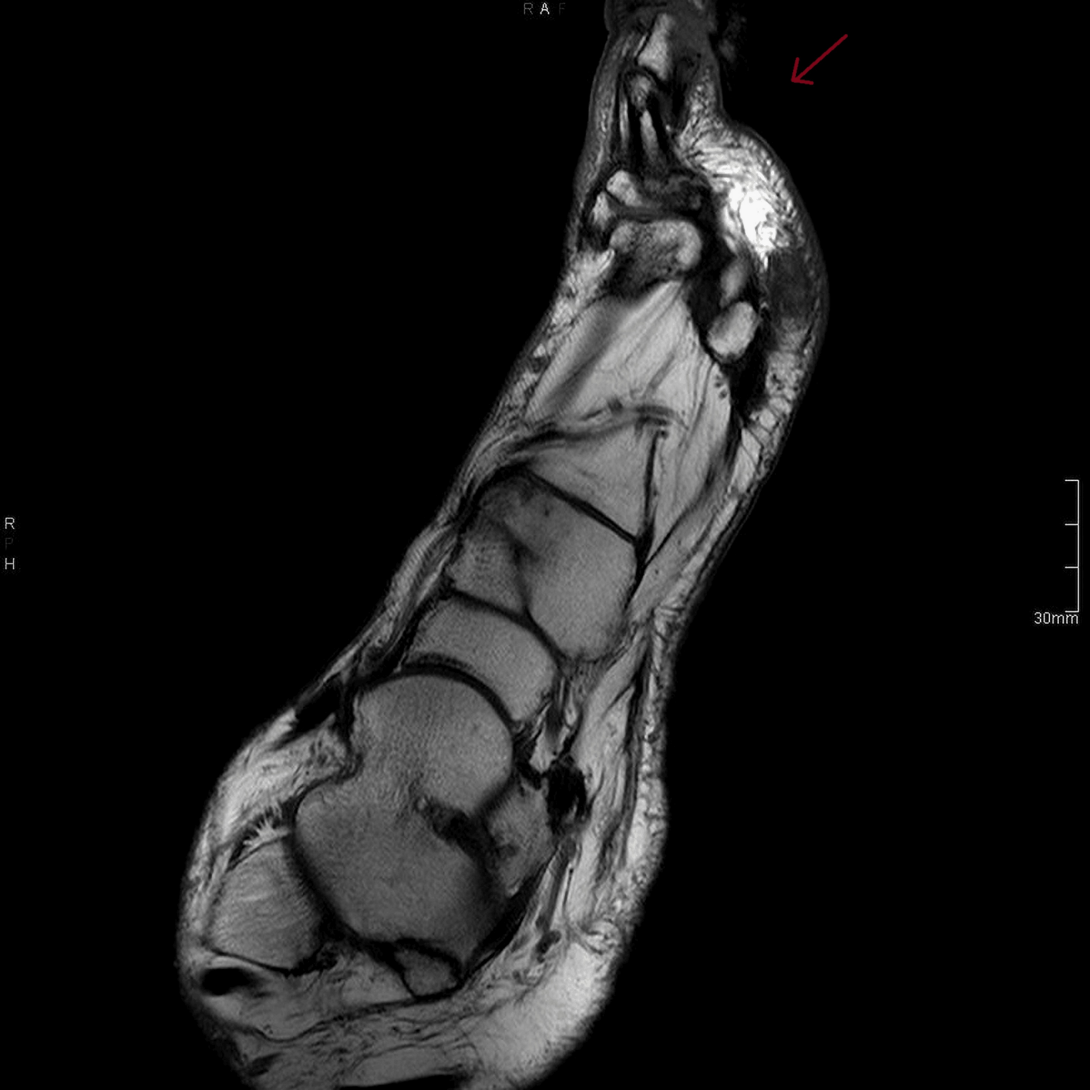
MRI foot right. MRI foot right reveals the destruction of the distal phalanx in keeping with osteomyelitis (red arrow).
